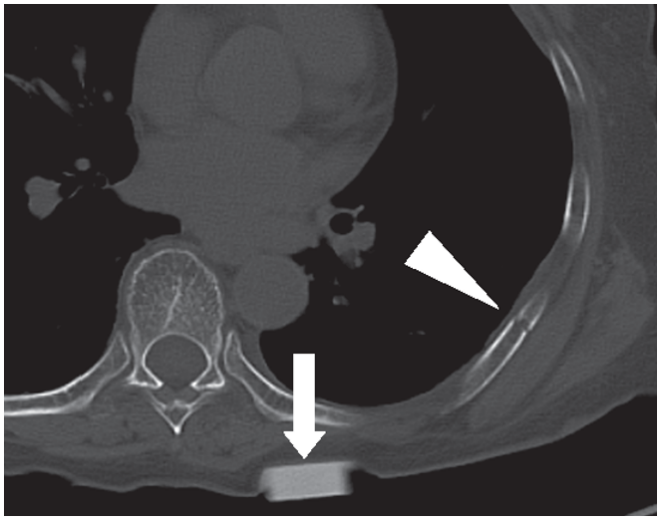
Computed tomography scan of the chest revealed correspondence of rib fracture (arrowhead) and pain location, marked with an eraser (arrow)
